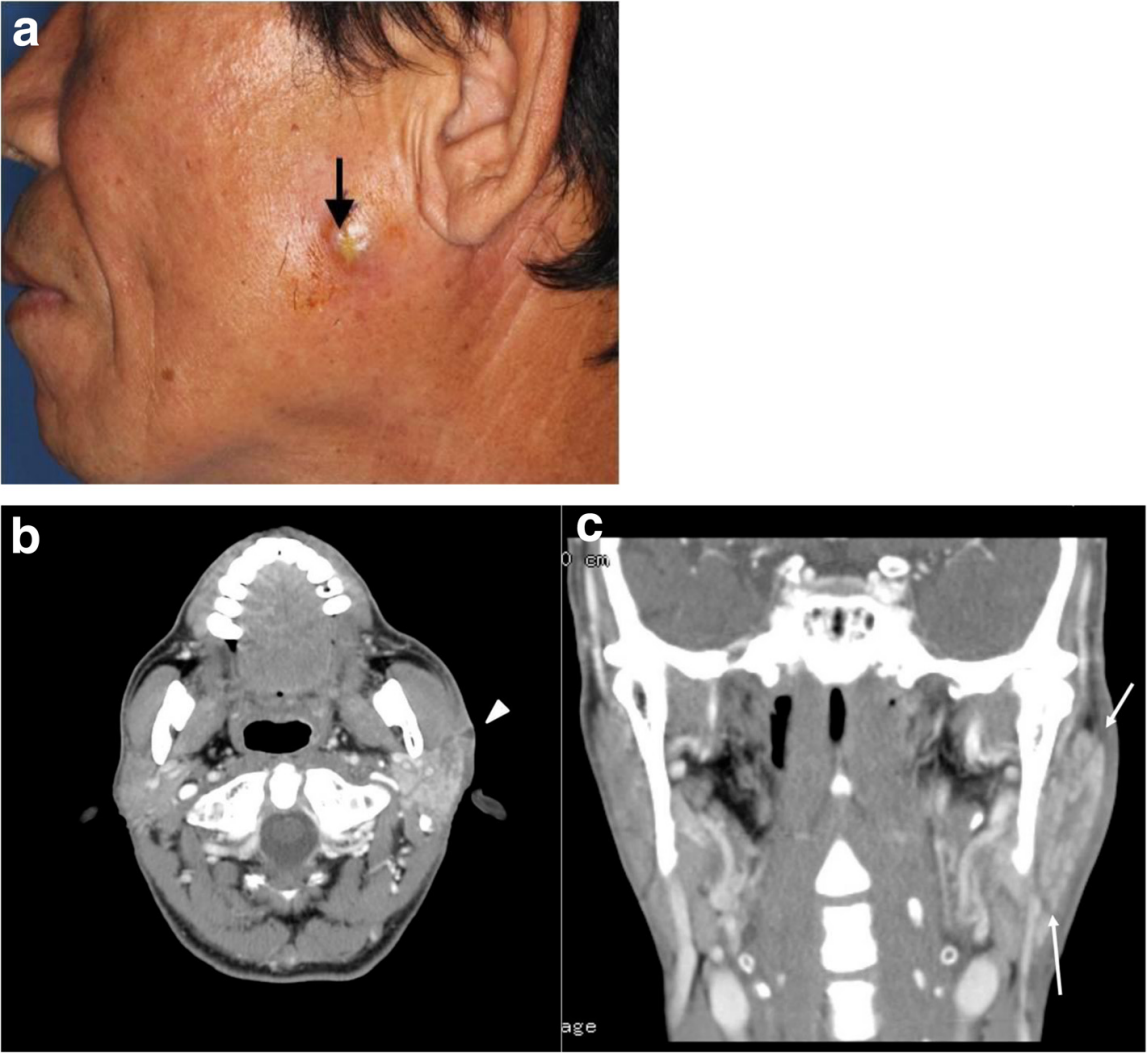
Clinical and mandible CT images of the case 2 patient (3 months after first visit). Cutaneous fistula was shown on the skin of right cheek (a). The fistula (b, *arrow head*) with enlarged enhanced mass (c, *arrow*) was found on mandible CT
